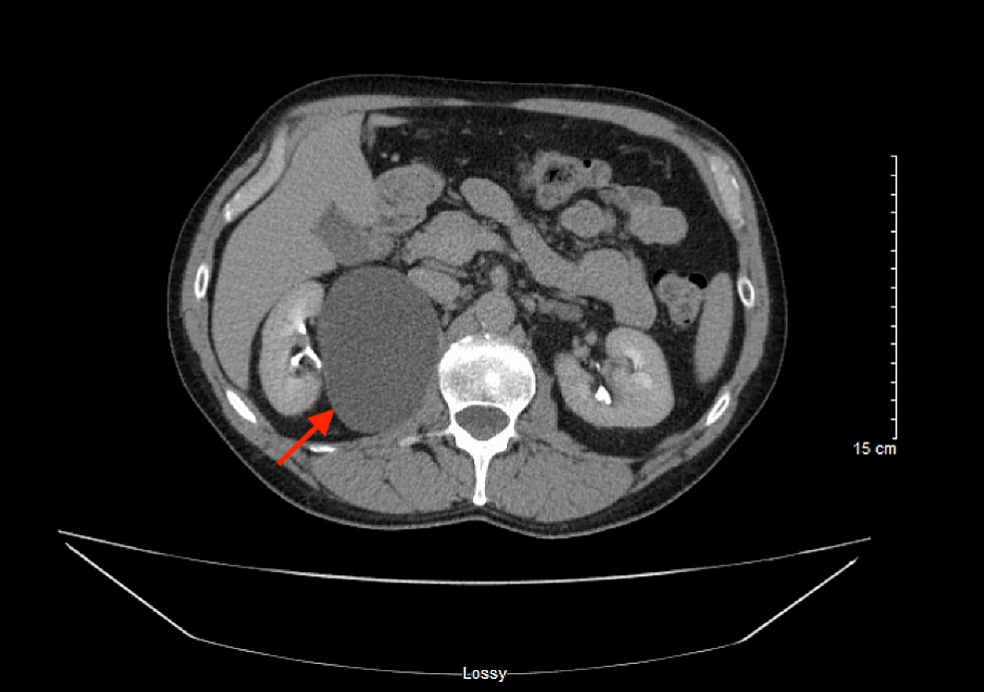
Axial CT scan image showing a cystic mass (red arrow) at the medial aspect of the right kidney measuring 8.8 cm in anterior-posterior dimension and 7 cm in medial-lateral dimension. CT: computed tomography
